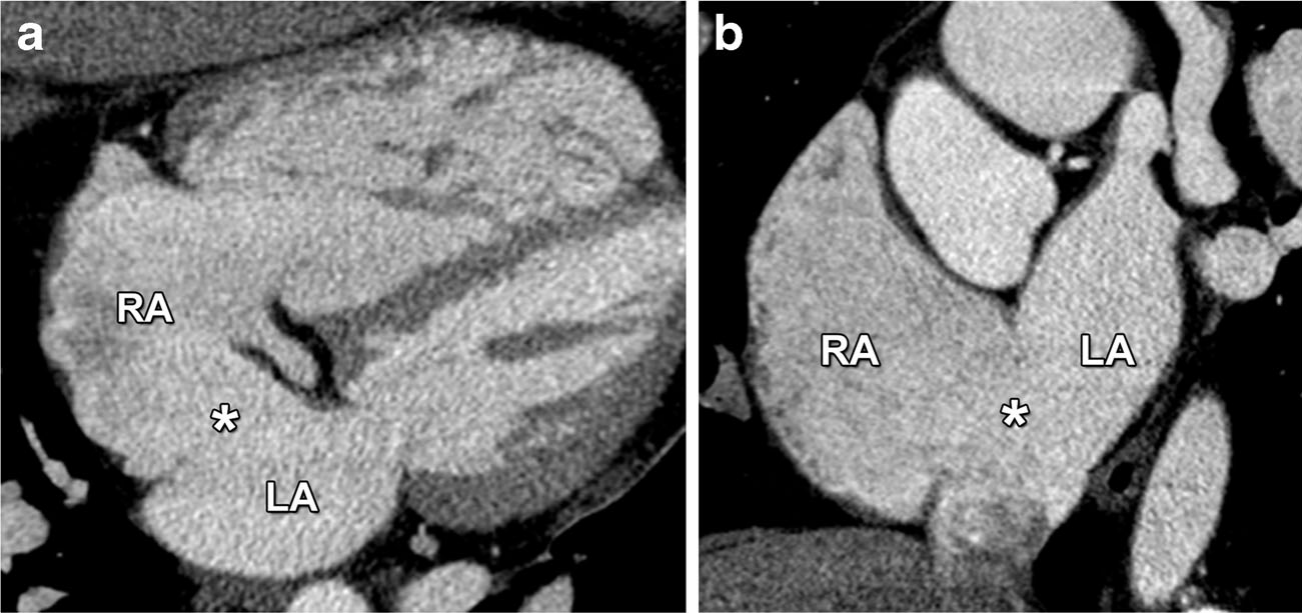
Four-chamber view (a) and short-axis (b) contrast-enhanced CT images showing a large OP defect (*asterisk*) allowing a communication between the left atrium (LA) and right atrium (RA). These defects are located in the part of the interatrial septum adjacent to the AV valves. Because OP defects are often large and lack a septal flap as in a patent foramen ovale, a shunt-induced volume overload with subsequent enlargement of the RA and ventricle can be seen
